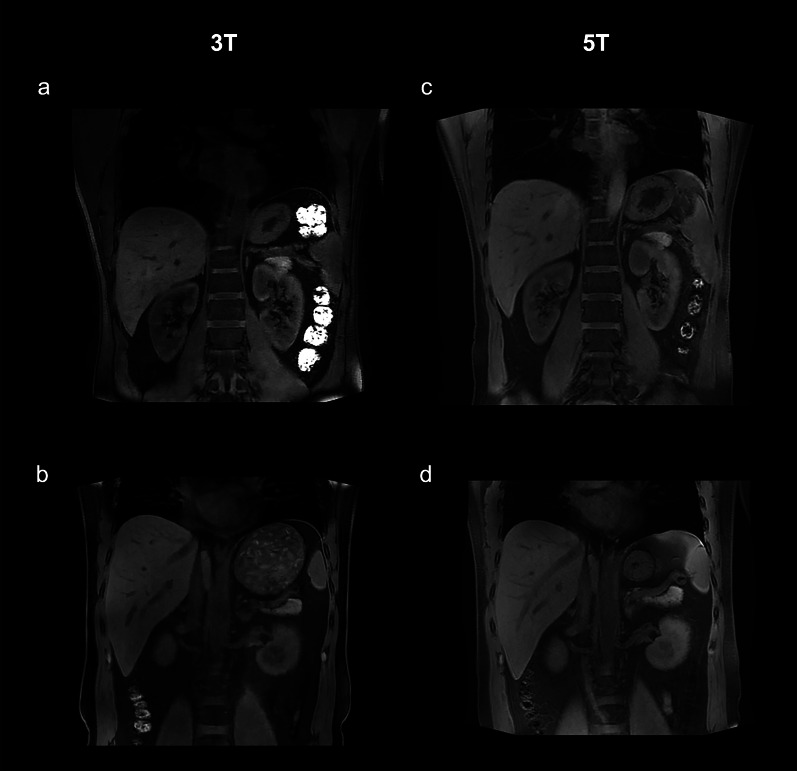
T1-weighted images of a 46-year-old male. a, b Coronal, breath-hold T1-weighted gradient-echo (GRE) sequence at 3 T; c, d Coronal, breath-hold T1-weighted GRE sequence at 5 T
